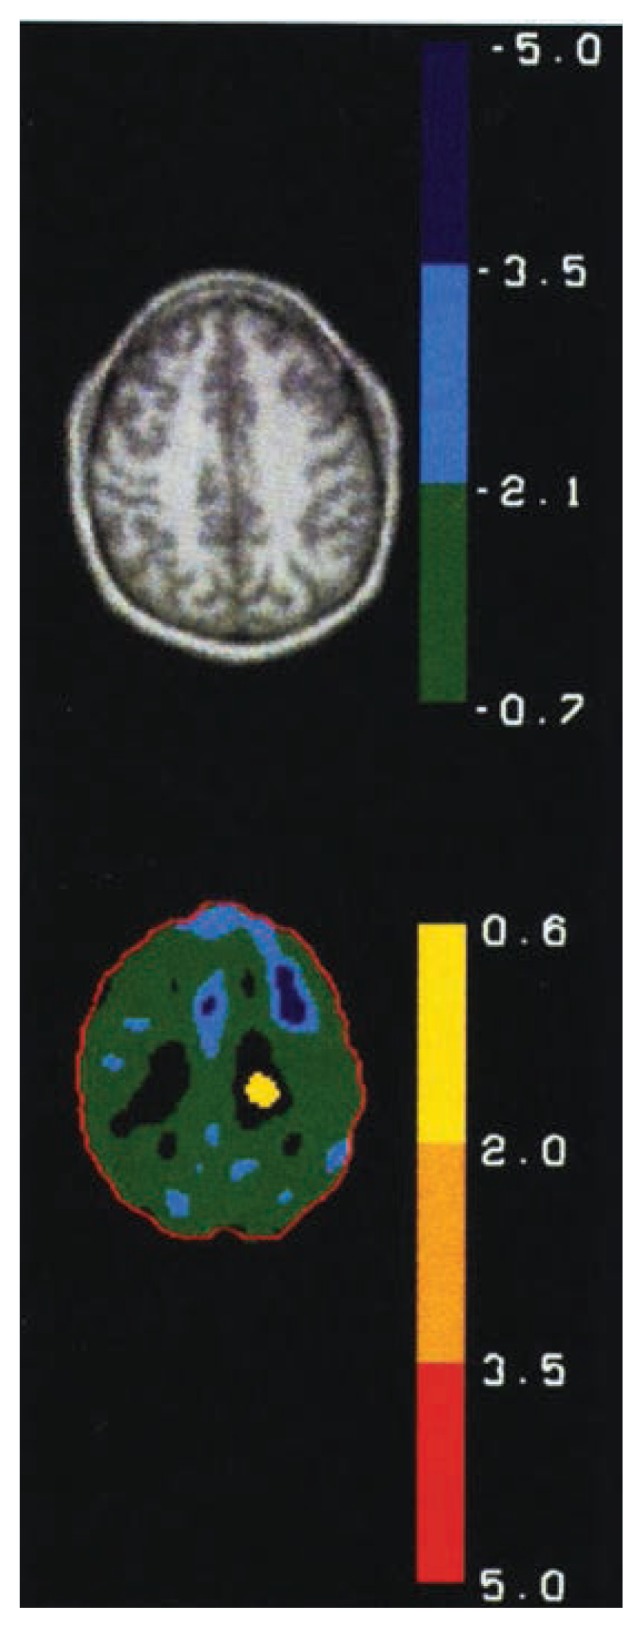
An axial slice (i.e., an image taken along a horizontal plane) from a magnetic resonance imaging-generated picture of the brain. In the lower image, the same slice is shown as a compilation taken from positron emission tomography (PET) scans of 19 detoxified alcoholics and 10 nonalcoholic volunteer males. The PET image shows differences between the alcoholics and the volunteers in the brain’s glucose metabolism. The dark blue regions are areas in which the alcoholics exhibited significantly lower glucose use than the volunteers.
